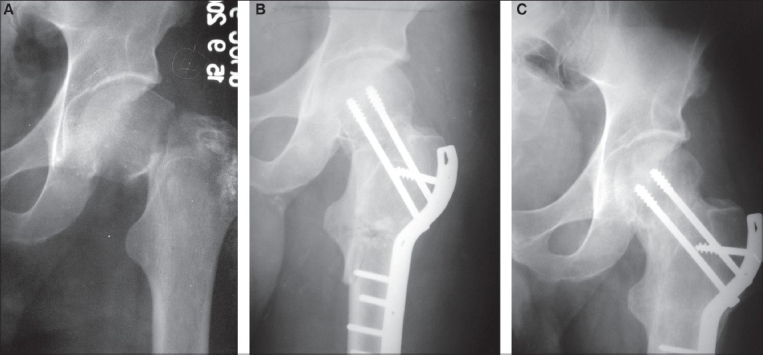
(a): Preoperative X-ray antero posterior view showing Garden III intracapsular femoral neck fracture. (b): Postoperative X-ray antero posterior view showing osteosynthesis of femoral neck fracture with two 6.5 mm partially threaded cancellous screws and primary valgus osteotomy fixed with contoured broad DCP. (c): X-ray ante antero posterior view at two-year follow-up showing sound union. Patient had normal function of hip and excellent results
